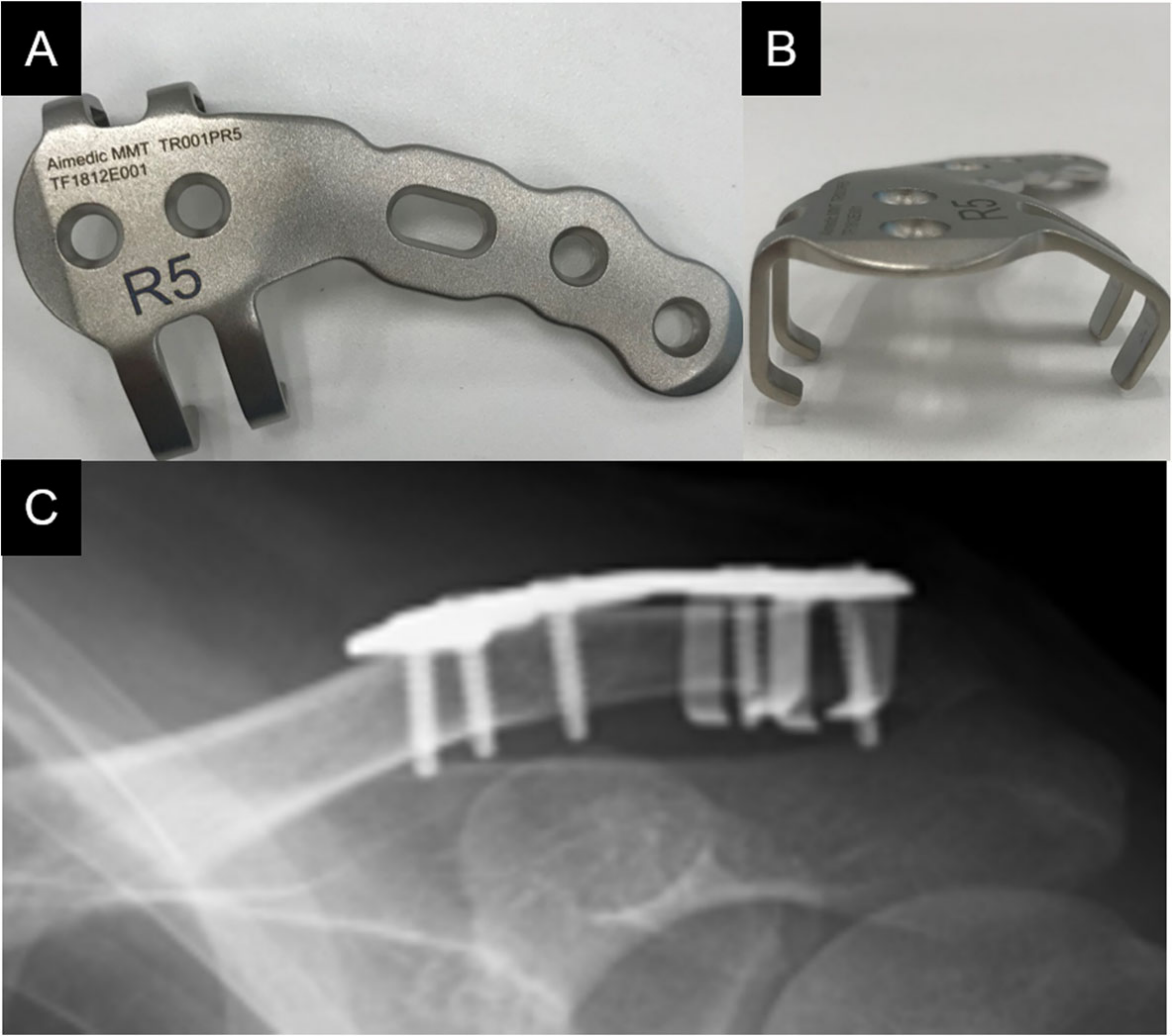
The photo images demonstrate a SCORPION® NEO plate (a and b). Plain radiograph showing osteosynthesis using a SCORPION® NEO plate within six days after injury (c)
